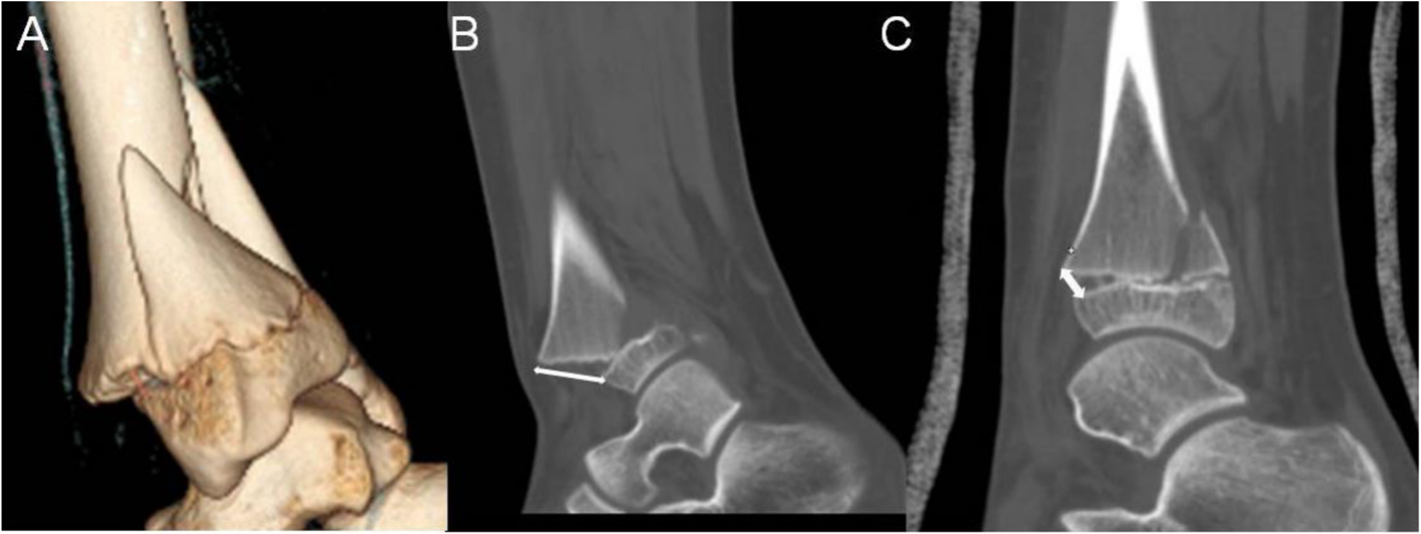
A 13-year-old boy fell downstairs and suffered a twist to his right ankle. a 3D CT view showed a displaced S-H II distal tibia fracture. b The maximal distance of displacement of the fracture was 18 mm (white double arrow) demonstrated in sagittal view. c The residual displacement was approximate 5 mm after closed reduction and casting
